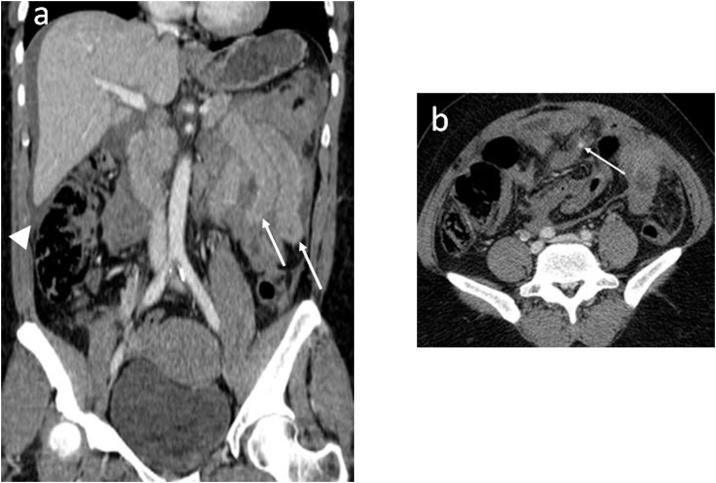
Coronal (a) and axial (b) iodinated contrast-enhanced CT images acquired in a 54-year-old woman after a traffic road accident show haemoperitoneum without solid organ laceration (a, arrowhead), non-focal jejunal bowel wall thickening (a, arrows), and mesenteric vascular extravasation (b, arrow), suggesting sBBMI. This was confirmed by immediate laparotomy (mesenteric suture, small bowel suture). McNutt and Faget scores were 1 (negative) and 10 points (positive), respectively.
